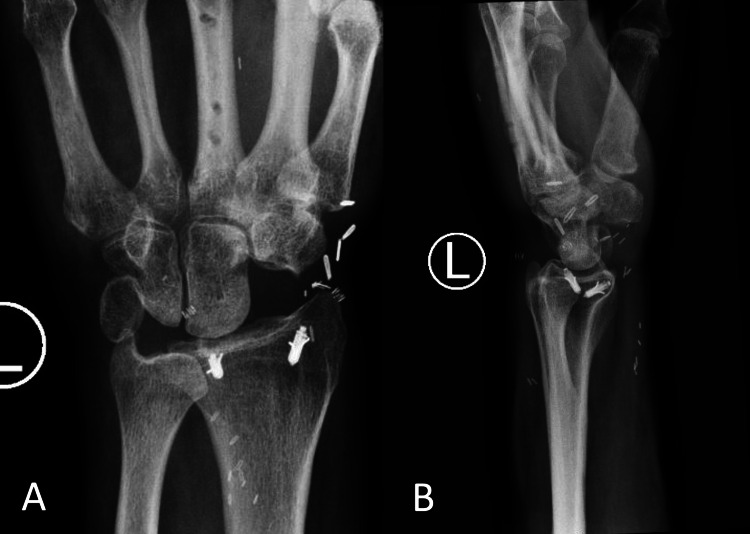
(A, B) Radiographs of the left wrist six months after replantation with proximal row carpectomy and 1.5 months after the removal of the dorsal spanning plate, demonstrating stable alignment
